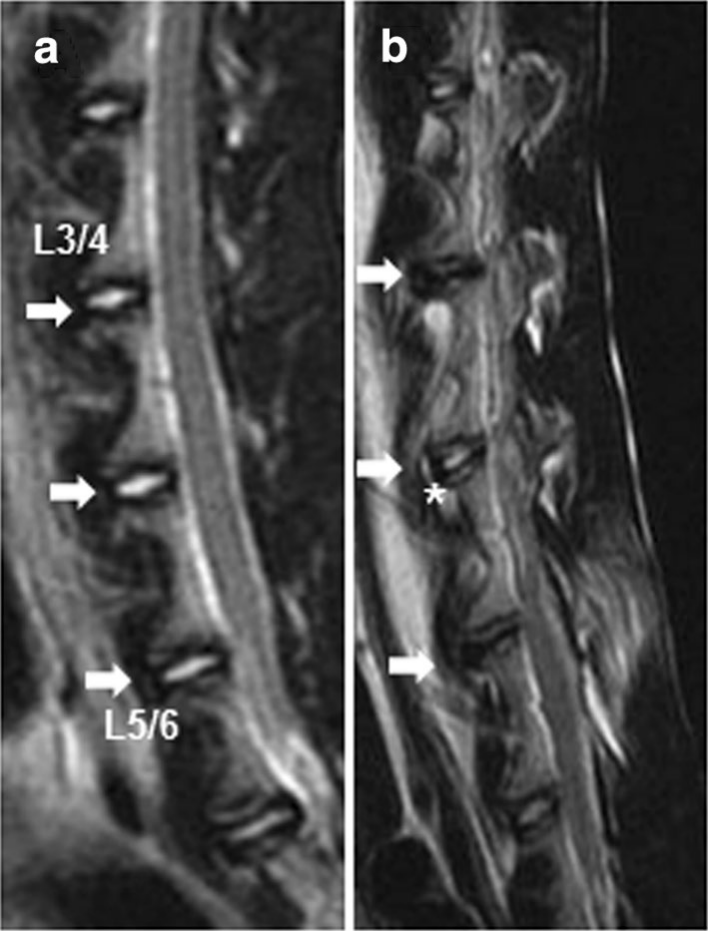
Representative magnetic resonance image (*MRI*) of the discs before surgery (a) and after surgery (b). Significant low T2-weighted signal intensity was clearly visible at the three experimental levels after surgery. The *white asterisk* indicates the partial loss of T2 signal intensity. The *arrows* indicate the operated disc
